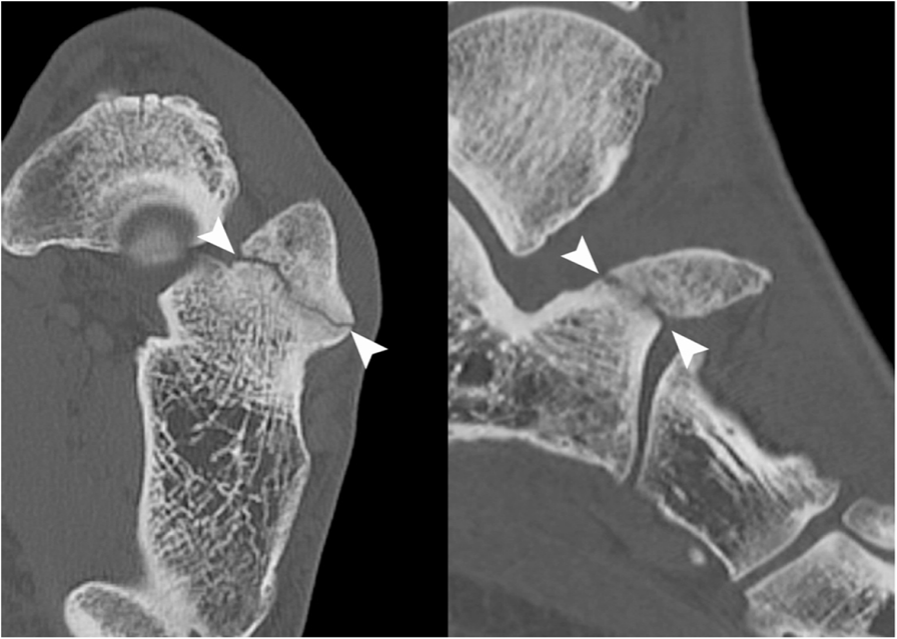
Axial (left) and sagittal (right) CT images. The arrowheads demonstrate the synchondrosis as a lucent line, that could be mistaken as a fracture
